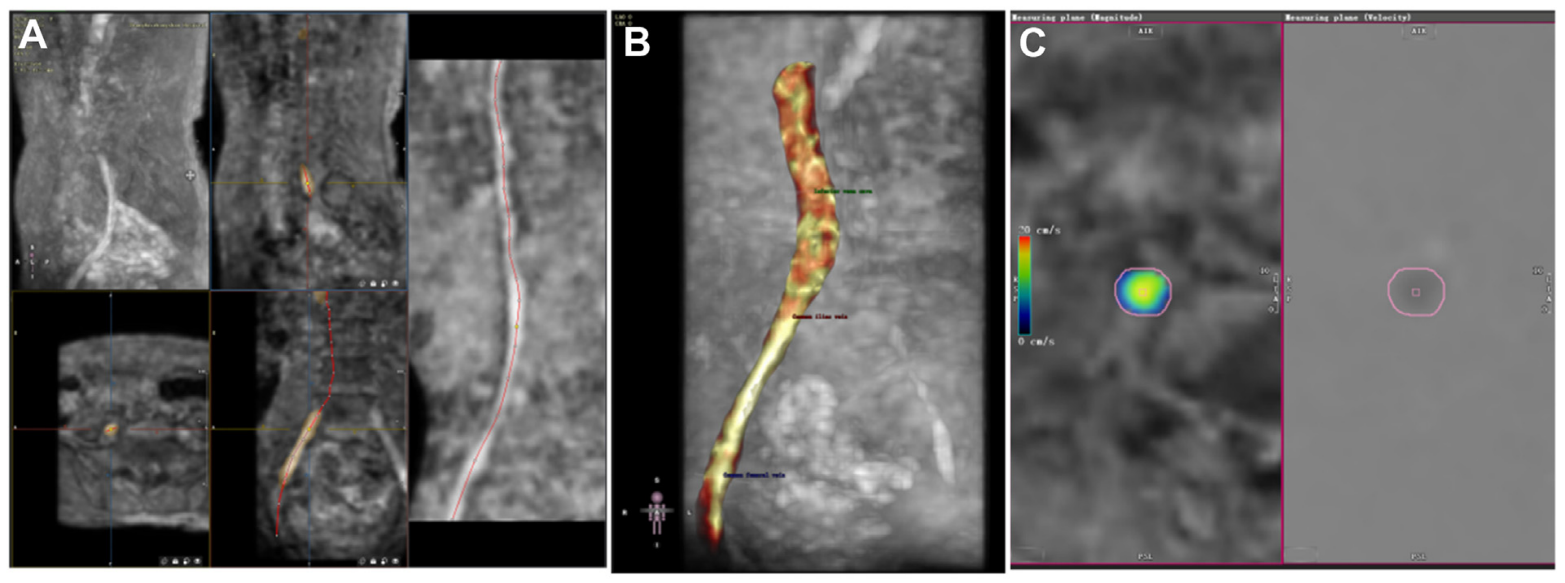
The data processing of four-dimensional flow magnetic resonance imaging (4D-Flow MRI), including determining the center line of lumen and adjusting the contours (A), the plane of measurement (B), and adjusting the region of interest (ROI) of measurement (C).
